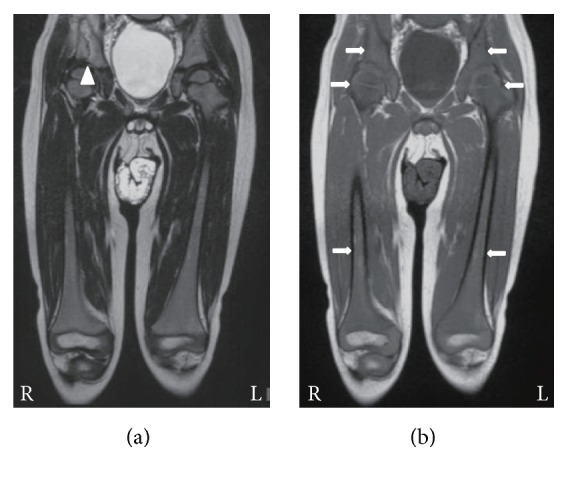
MRI findings in case 3. At 3 days after the onset, (a) T2-weighted image depicts high signal intensity in the right ilium (arrowhead) and (b) T1-weighted images reveal diffuse low signal intensity in the bilateral iliac and femoral bone marrow (arrows).
